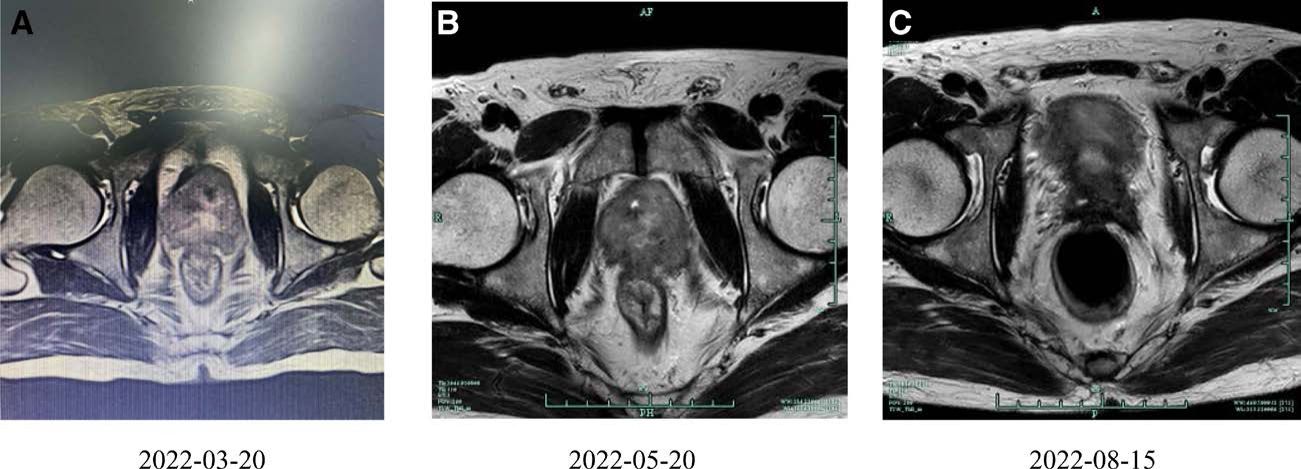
MRI of prostate disease at baseline and course of treatment. A)MRI results of baseline on 2022-03-20. Size of prostate: 5.3*6.0*5.7cm B) MRI results after 2 courses of bicalutamide and 3 courses of docetaxel on 2022-05-20. Size of prostate: 4.7*4.5*5.2cm. C) MRI results after 4 courses of zimberelimab on 2022-08-15. Size of prostate: 2.8*3.0*3.6cm. MRI = magnetic resonance imaging.
